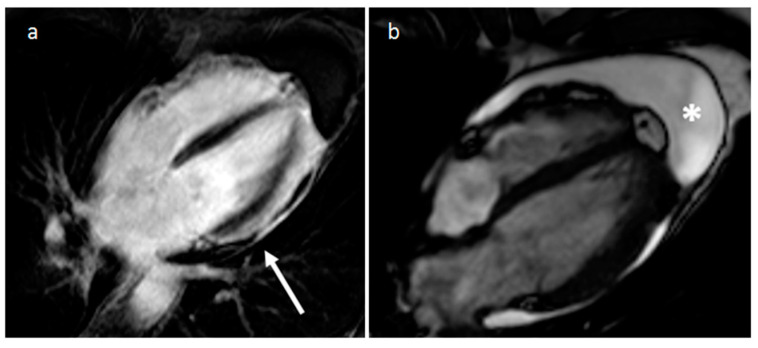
Parietal pericardium thickening (white arrow) and enhancement on late gadoliunium enhancement sequence (a) and cine MR images (b), with associated pericardial effusion (*).
